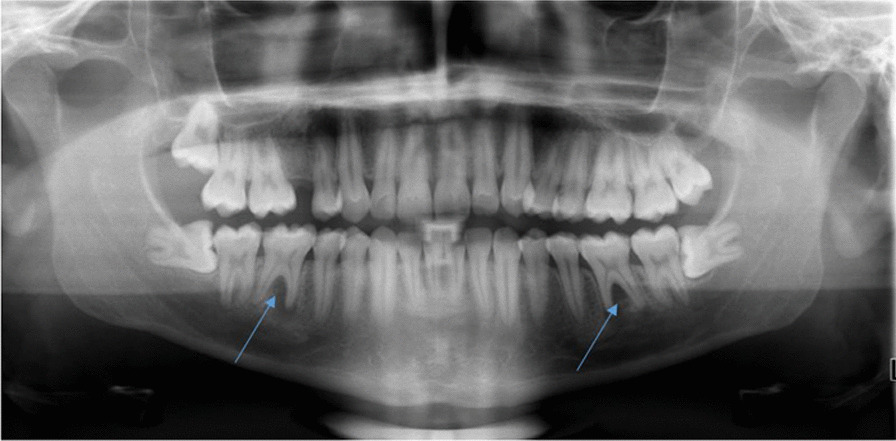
X-Ray taken in January 2020 by the primary dentist. Apical and interradiuclar osteolysis on tooth 36 and 46. No signs of caries and no fillings are visible
